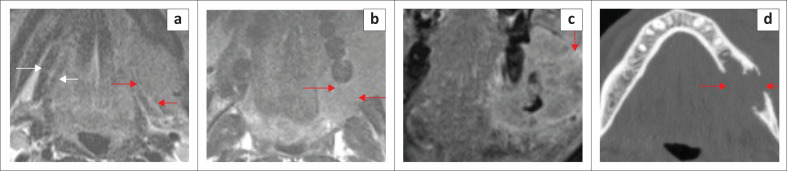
Stage T4 squamous cell carcinoma of the mandible in an adult patient. There is a large bulky mass lesion involving the left mandibular body, with full-thickness osseous destruction, and the lateral floor of the mouth, with invasion of the left mylohyoid and hyoglossus muscles (red arrows on the left and normal muscles on the right (white arrows) in (a, b; T2-axial views) and on the coronal T1 contrast-enhanced fat-suppressed image (c). There is extensive infiltration of the left masseter muscle (c) and retromolar trigone (b, red arrows). CT mandible (d) shows full-thickness cortical osseous destruction on the left.
